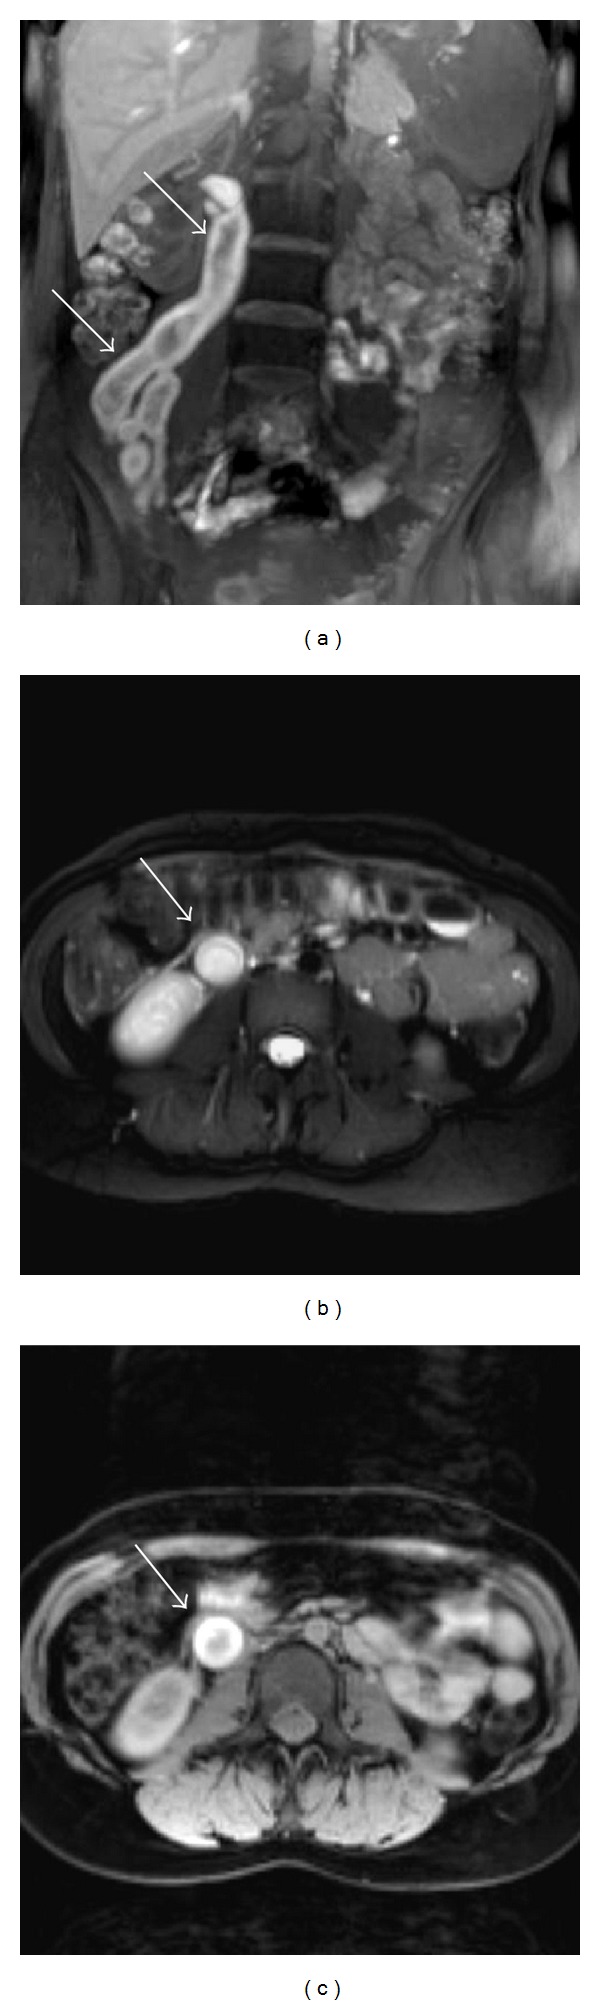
MR images show subacute right ovarian vein thrombosis. Coronal T1-weighted image (a) demonstrates an enlarged right ovarian vein showing increased signal (arrow) with an isointense central focus and axial T2-weighted image (b) shows high signal (arrow) indicative of methemoglobin within a complex thrombus. Contrast-enhanced axial T1-weighted image (c) reveals significant contrast enhancement of the vessel wall.
